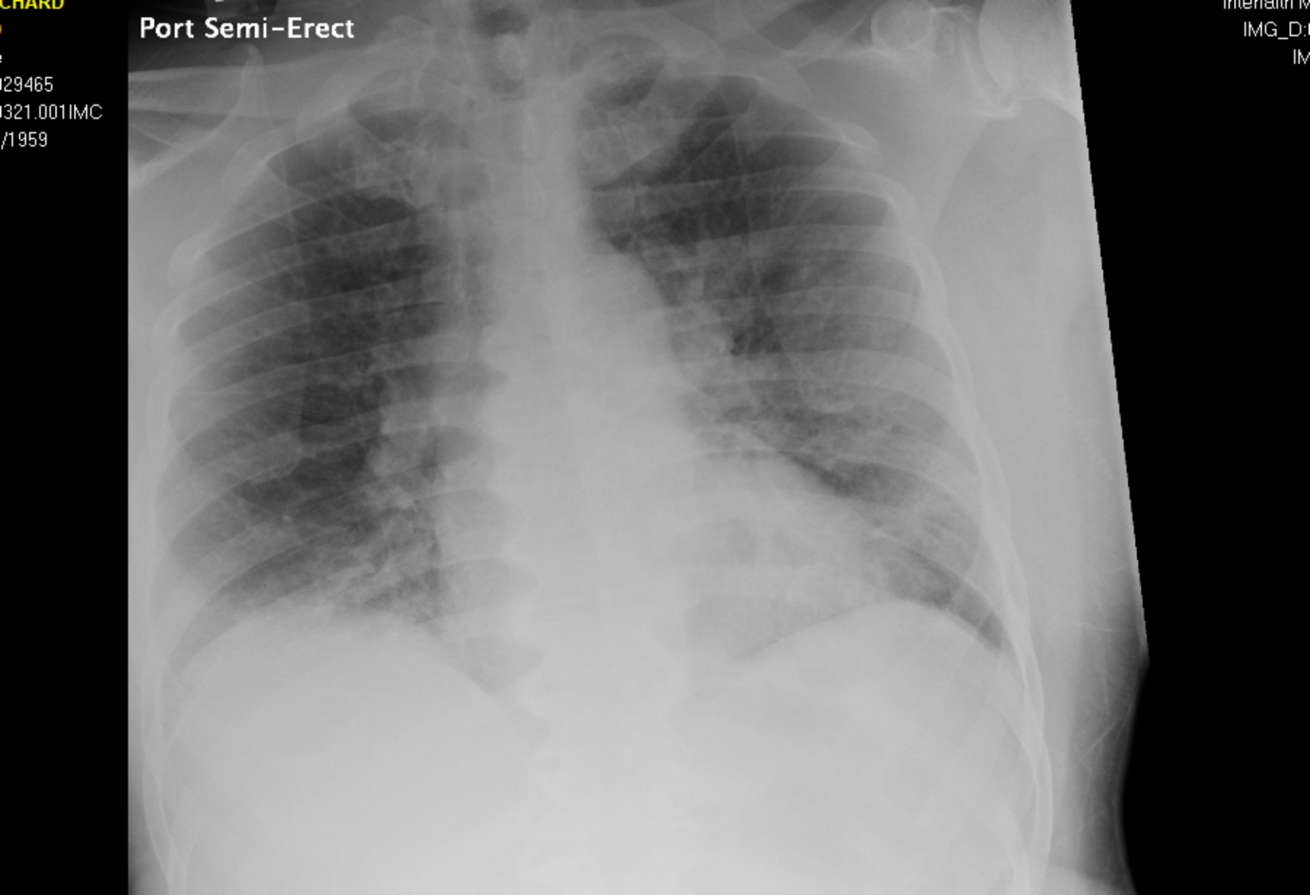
Case 2 chest X-ray on admission April 03, 2020
